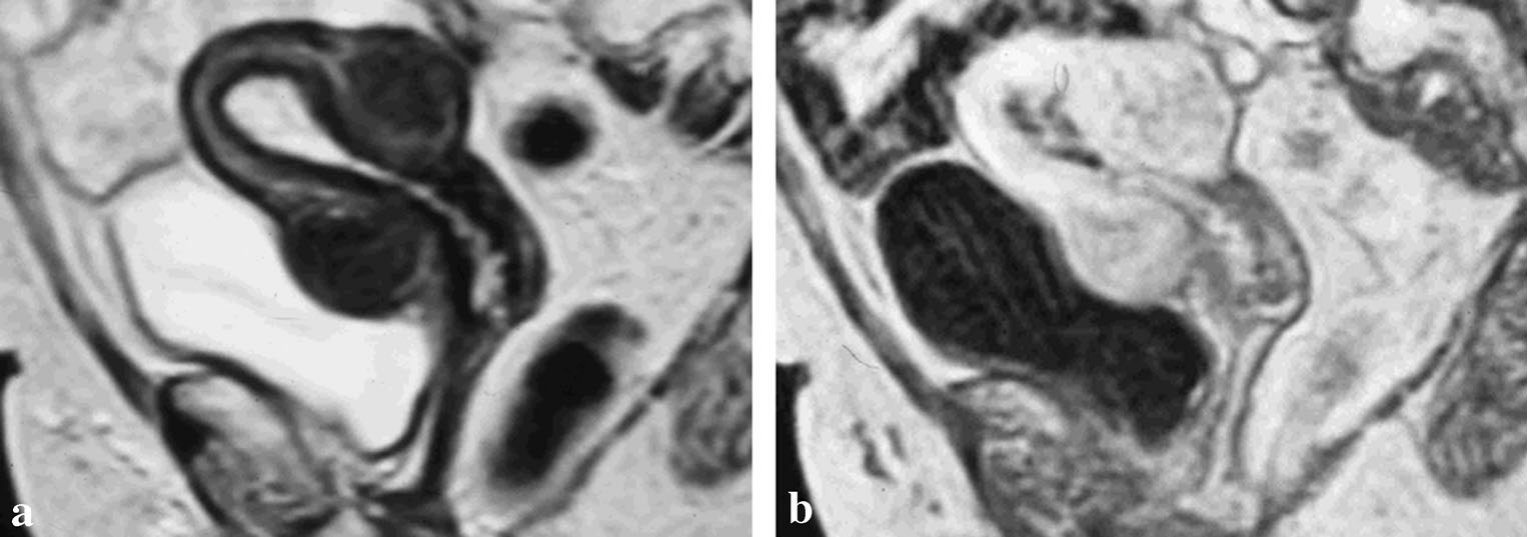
a, b. High-grade endometrioid carcinoma within an endometrial hyperplastic polyp in a 62-year-old woman with Cowden syndrome. T2-weighted image (a) shows distension of the uterine cavity due to a polyp, more evident on post-gadolinium FS T1-weighted image (b), which is hypoenhancing compared with myometrium. Normal uterine zonal anatomy is preserved. Note the bosselated and smooth outer contour of the uterus due to leiomyomas
